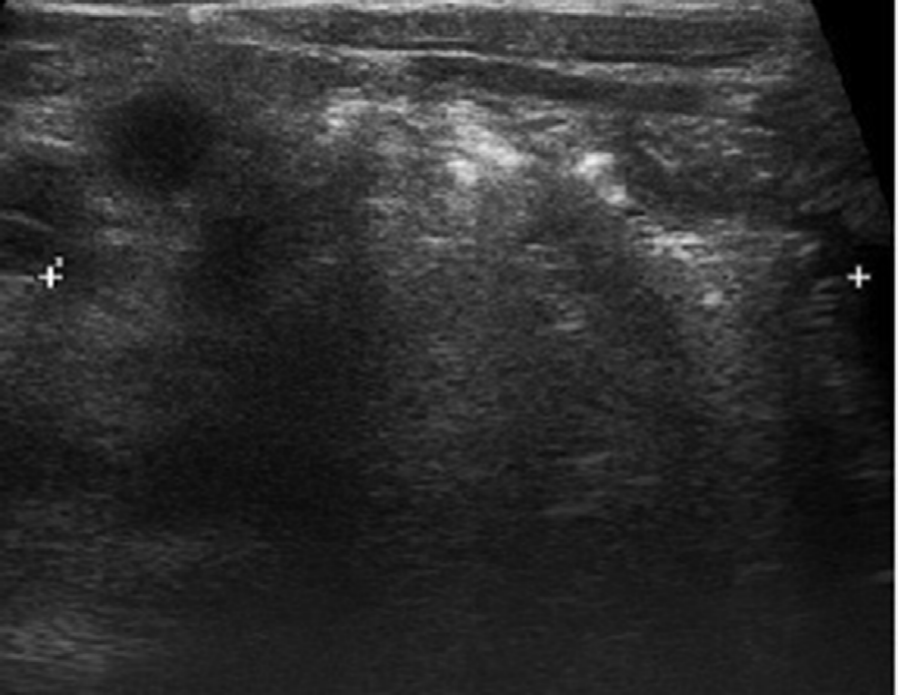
Ultrasonografic view of inguinal hernia sac.
